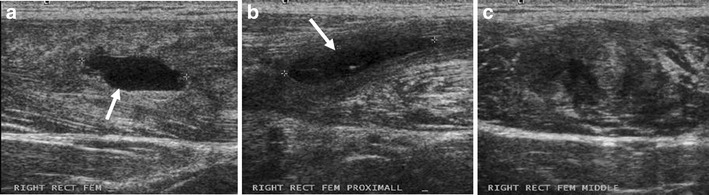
Ultrasound images of a 20-year-old football player (the same patient as the one shown in Fig. 4) taken at the same time as Fig. 4a–c. a A newly developed haematoma (*arrow*) measuring 2 cm × 0.8 cm is noted in the proximal mid-portion of the rectus femoris muscle. b More proximally, a known pre-existing fluid collection (2.5 cm × 0.5 cm, *arrow*) from the previous injury 3 weeks prior is observed. It is difficult to differentiate (a) as a new and (b) as an older lesion on the basis of ultrasound alone. c In between these two lesions, a notable echo inhomogeneity is noted, suggestive of a partial tear of the proximal rectus femoris muscle
